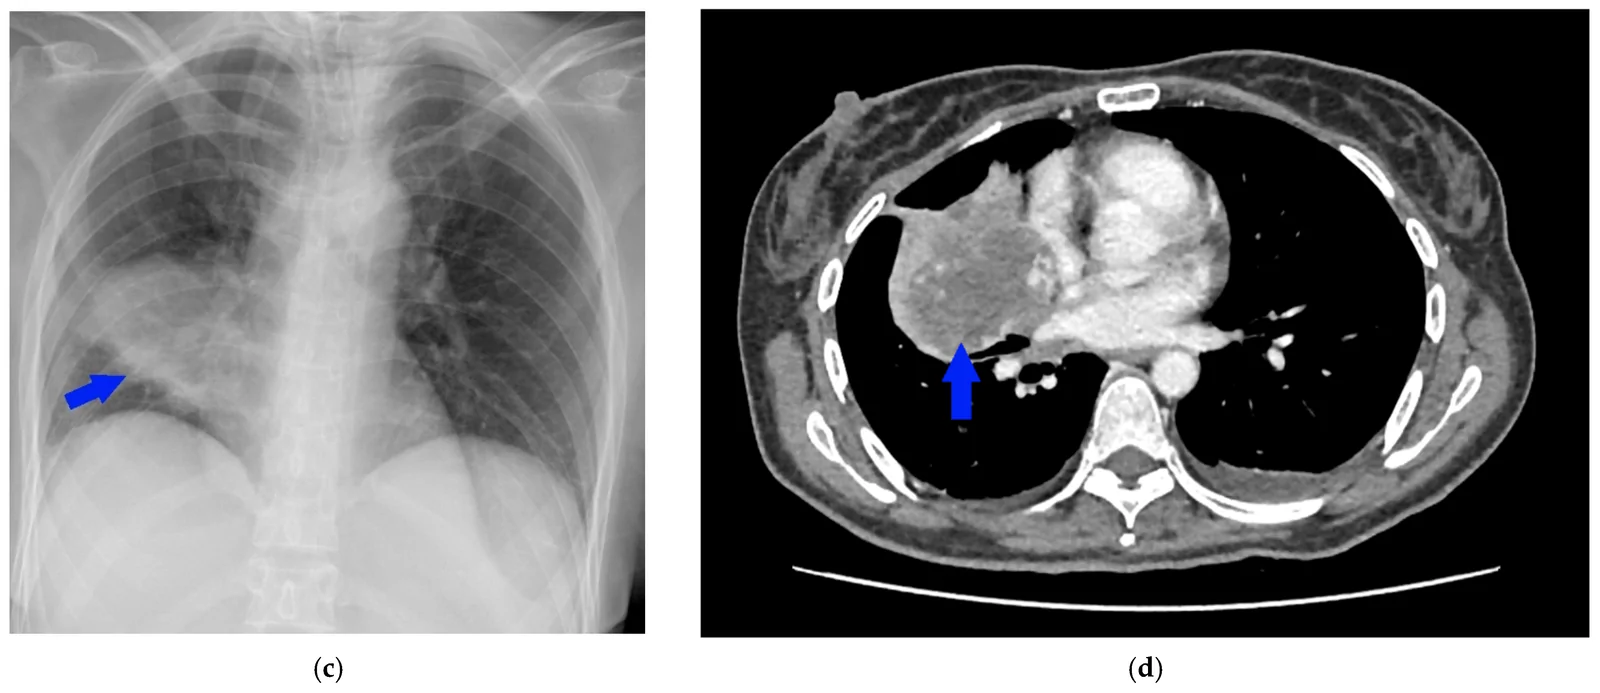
During hospitalisation, chest X-ray (c) and CT (d) scans revealed a 58 × 70 × 65 mm mass in the right middle lobe of the lung—marked with blue arrow. Due to deterioration of the patient’s condition and technical difficulties, a biopsy of the lung lesion was not performed.
